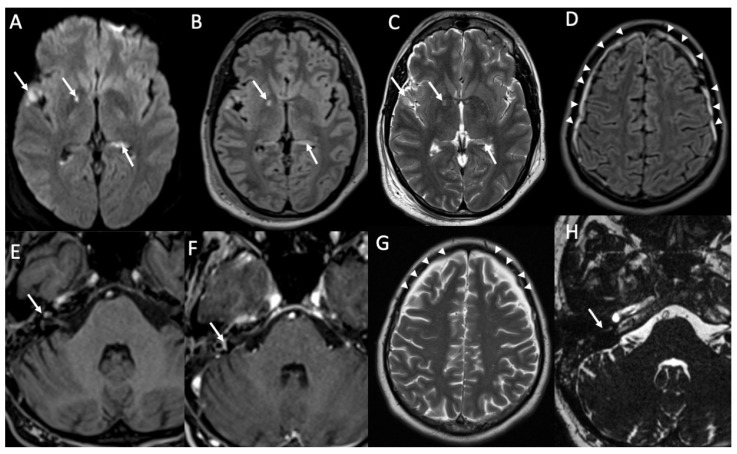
Axial DWI (A), FLAIR (B), T2 (C,G), enhanced FLAIR (D), T1 with fat-suppression (E), enhanced T1 with fat-suppression (F) and axial 3D-CISS show multiple recent ischemic lesions (arrows in A–C) and pachymenangeal enhancement (arrowheads in D) in a patient with Streptococcus pneumoniae meningitis. There is also cochlear hemorrhage on the right side (arrow in E), with enhancement of the structures of the inner ear (arrow in F). Axial T2 (G) and 3D-CISS (H) performed after one month depict subdural hygroma in the frontal regions bilaterally (arrowheads in G) and loss of the normal fluid signal of the internal right ear due to ossificans labyrinthitis (arrow in H).
